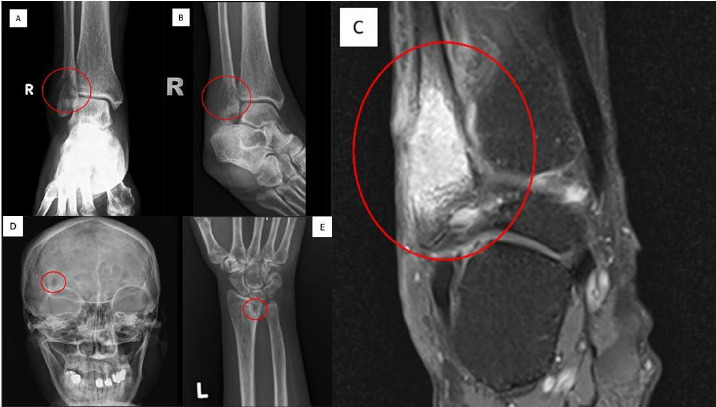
Plain radiographs of the right ankle in AP and mortise views (A and B) showing an aggressive lucent bone lesion with a pathological fracture at the distal fibula. Representative MRI scan in coronal view on T1 FS post-gadolinium sequence (C) demonstrating an enhancing right distal fibular lesion causing cortical destruction with surrounding; inflammatory changes.
